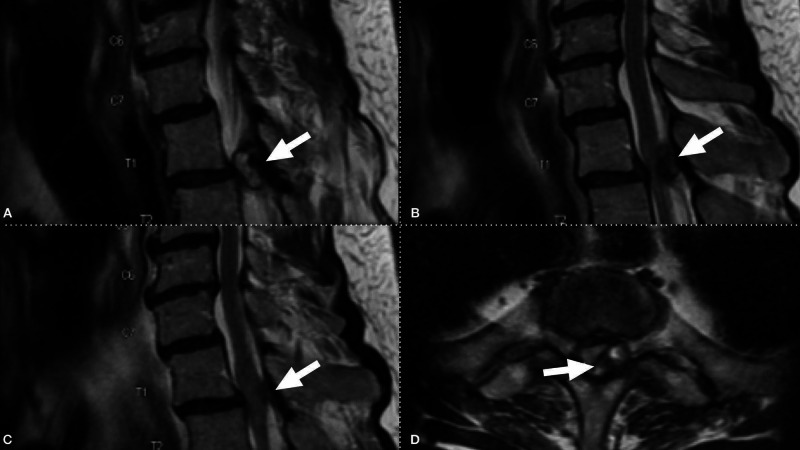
MRI of the thoracic spine demonstrating a large left T1-2 synovial cyst causing severe compression with associated T2 signal change within the spinal cord Cervical spine MRI demonstrating T1-2 synovial cyst, with severe spinal cord compression, see arrows demonstrating cyst and subsequent compression. (A,B) Sagittal T2 images demonstrating a large T1-2 synovial cyst causing spinal cord compression. (C) Midline sagittal T2 image demonstrating T2 signal changes within the spinal cord. (D) T1-2 level axial T2 images demonstrating severe compression from left-sided cyst, with the arrow demarcating the compressed and displaced spinal cord.
